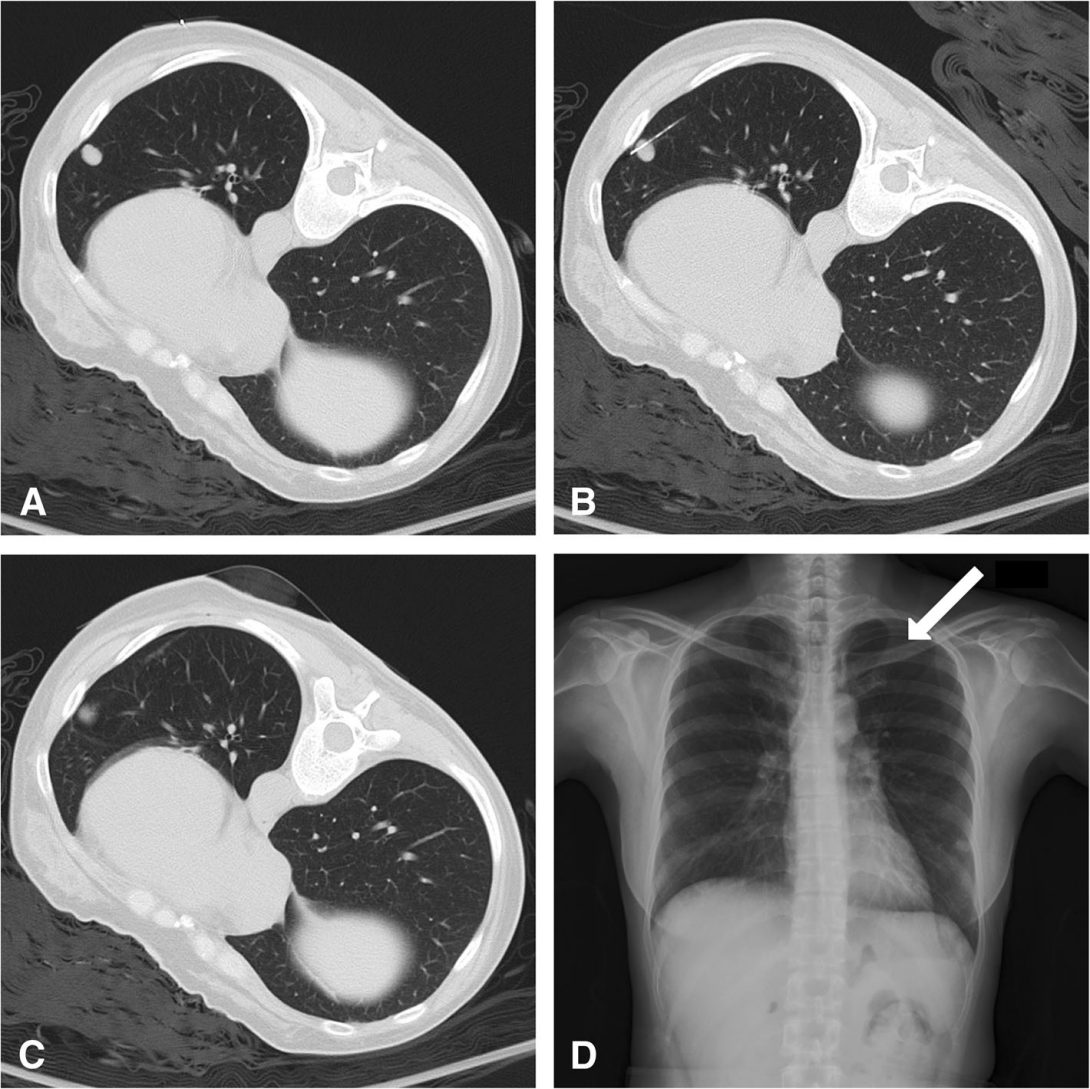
CT-guided pulmonary biopsy in a 45-year-old female. a Pre-biopsy CT with standard lung window revealed a 10-mm nodule over the left lower lobe. A metallic marker was placed over the skin for location. b The biopsy was performed using a 17-gauge introducing needle and 18-gauge cutting needle. To prevent the needle from crossing the fissure and being blocked by the rib, a proper entry site was chosen. The final position of the cutting notch of the needle was just inside the nodule. c Post-biopsy CT revealed minimal pulmonary hemorrhage around the needle path, and no pneumothorax was found. d A standing chest X-ray was performed 24 hours after the procedure. The pleural line (arrow) over the left upper chest was visible, and pneumothorax over the left side was confirmed
